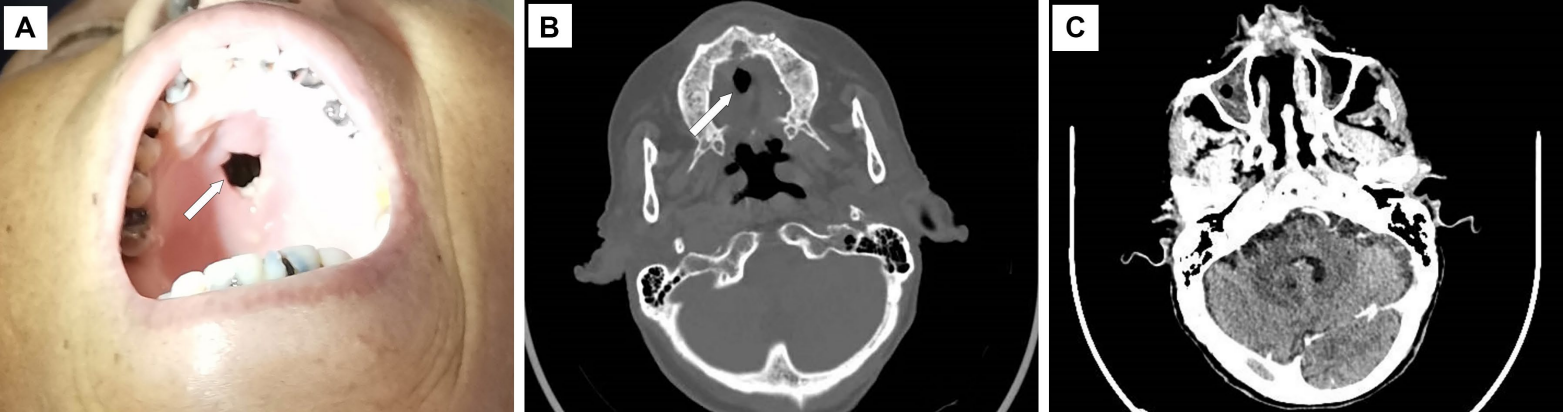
Upper palate defect and maxillofacial CT scan image. There is a defect of approximately 1 cm in diameter on the upper palate (A, B), and the CT scan shows the thickening and enhancement of the nasal mucosa (C). The white arrow indicates the defect.
